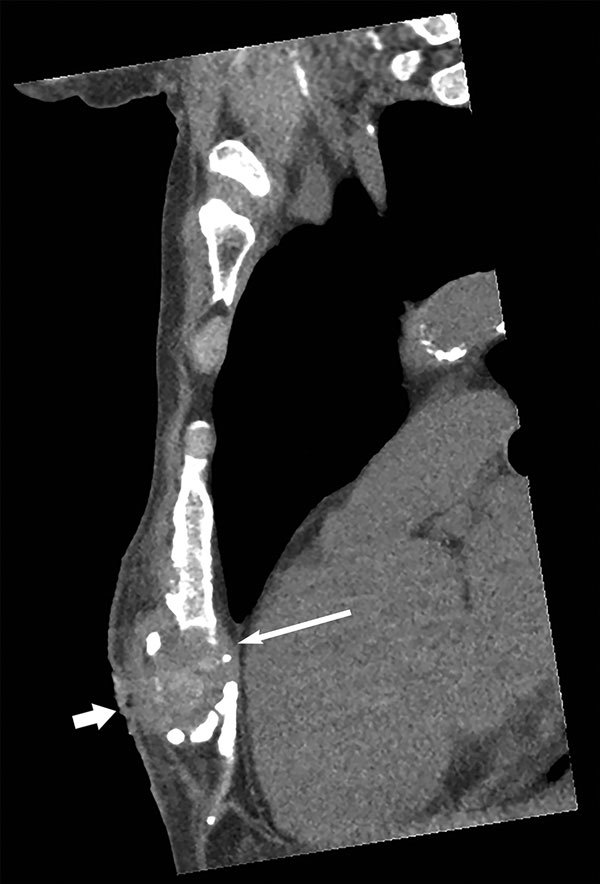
Figure. Computed tomography scan of the chest wall (sagittal section, bony windows) of man from Kenya with *Candida auris* sternal osteomyelitis, Australia, 2015. Image shows bony erosion and fragmentation of distal sternum (thin arrow), together with a 3.3-cm abscess and a sinus tract in the subcutaneous tissues (thick arrow).
